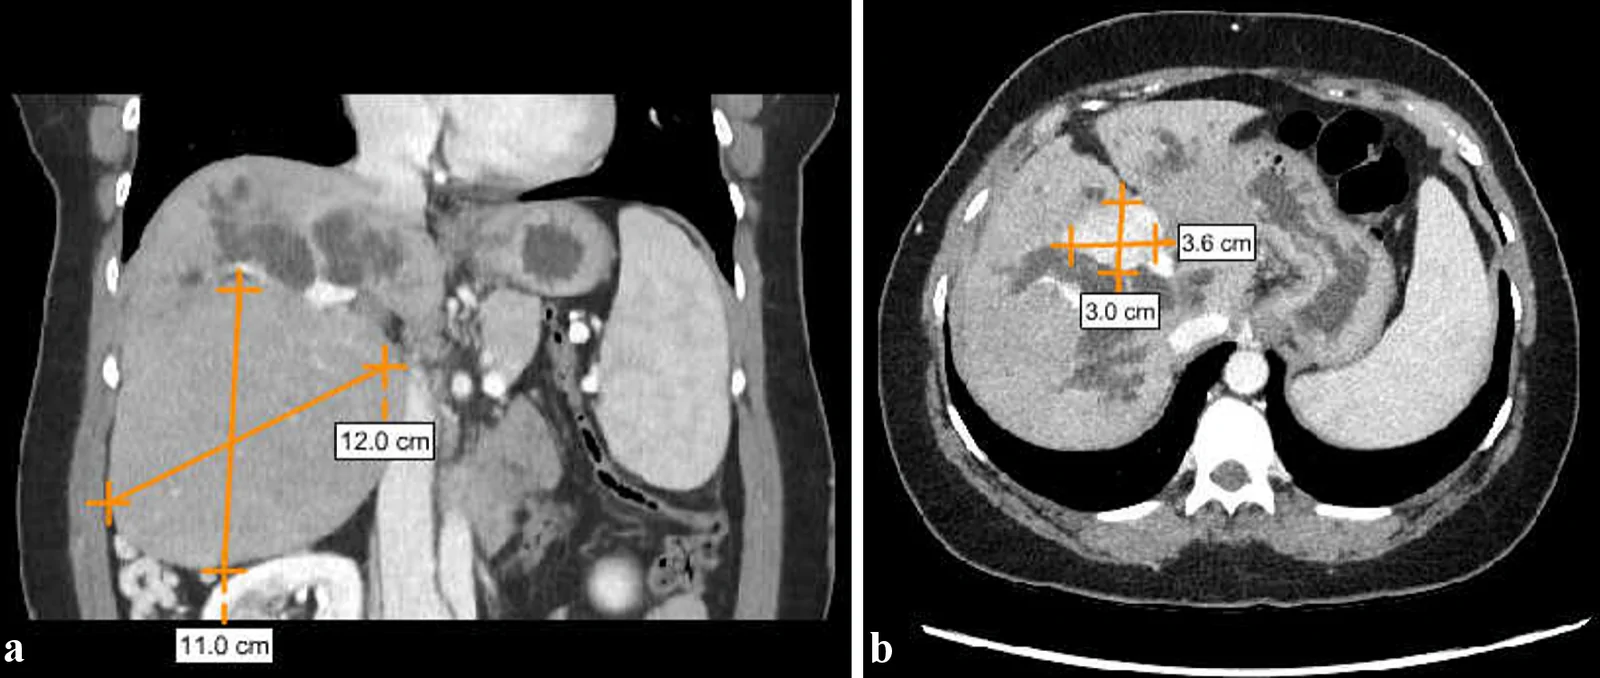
CT abdomen–pelvis with intravenous (IV) contrast enhancing, showing (a) markedly hypertrophied segment extending inferiorly from the liver (12 × 11 cm) and (b) a 3.6 × 3.0 cm tubular arterially enhancing structure anterior to the main portal vein. CT: computed tomography.
